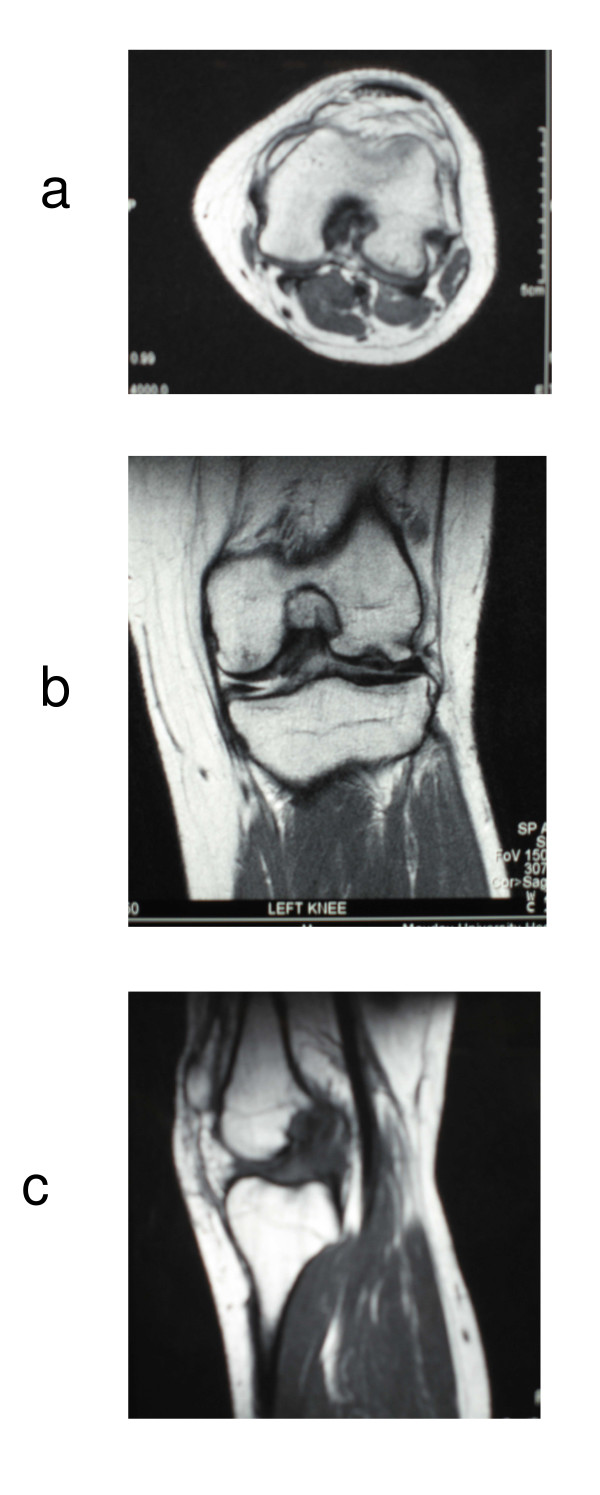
Magnetic resonance imaging of the right knee.
